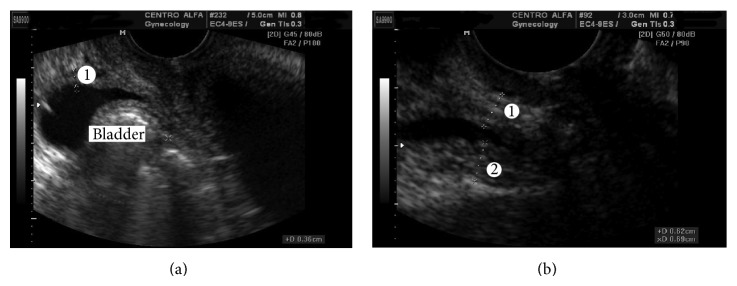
Two-dimensional transvaginal ultrasound. (a) Measurement of anterior wall thickness of bladder (1). (b) Measurement of thickness wall in trigone bladder (1); measurement of thickness wall in dome bladder.
